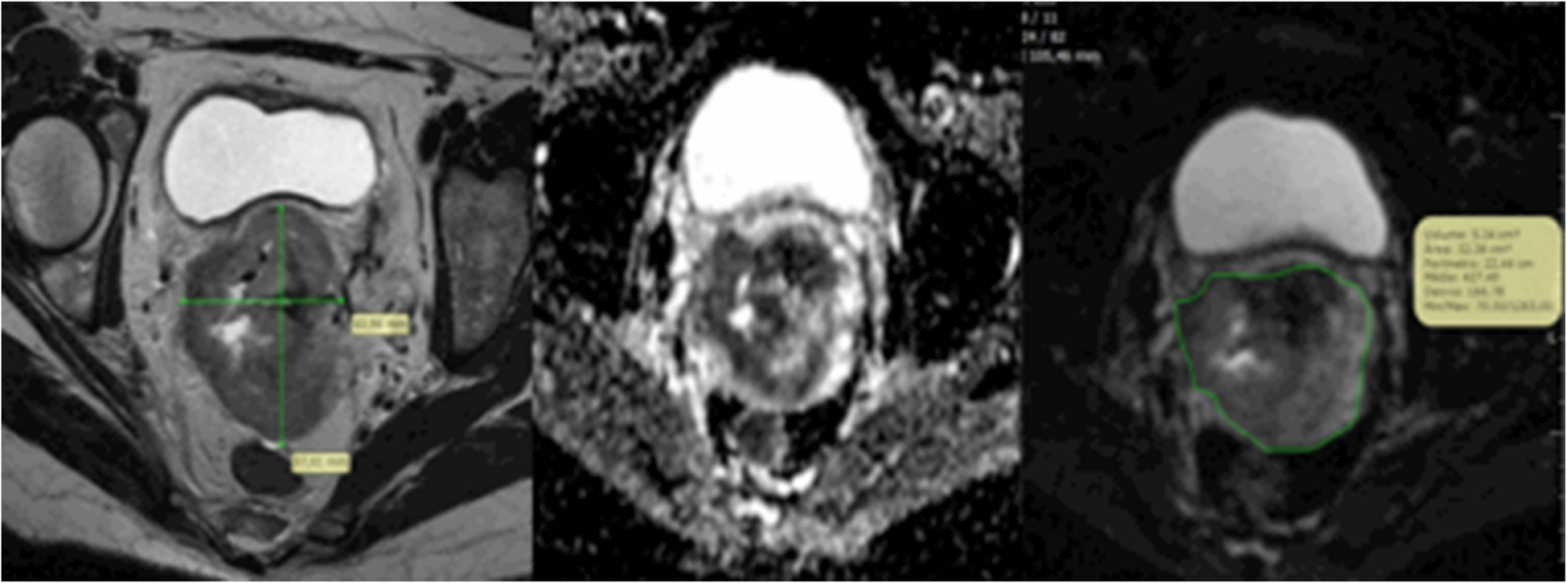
Magnetic resonance imaging examples of axial slices of: T2 weighted (*left*), diffusion weighted imaging (*center*), and region of interest drawn on an attenuation diffusion coefficient map (*right*)
